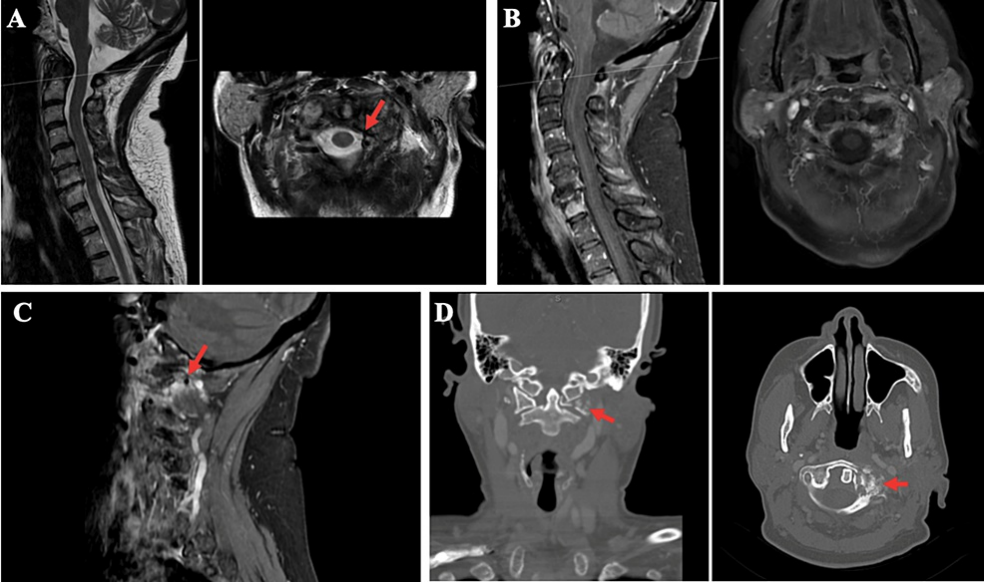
Sagittal (left) and axial (right) MRI of the patient A. T2-weighted and B. T1 fat saturation MRI sequences demonstrating C1 lateral mass lesion (red arrow) and displacement of the vertebral artery with no epidural tumor extension or cord compression. C. Parasagittal T1 sequence demonstrating lateral tumor extension with encasement of the vertebral artery (red arrow). D. Coronal (left) and axial (right) CT angiogram demonstrating osteolytic left lateral mass lesion encasing and narrowing the vertebral artery (red arrows).
